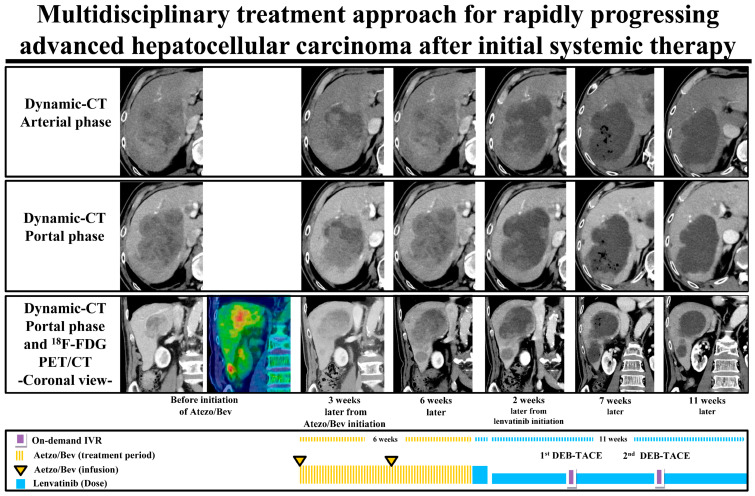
The flow of a multidisciplinary treatment approach for a patient with rapidly progressing advanced HCC after initial systemic therapy. The patient showed rapid progression of HCC after first-line Atezo/Bev therapy and received lenvatinib as second-line treatment. After initiation of lenvatinib, the tumor showed extensive necrosis and good tumor control in combination with subsequent DEB-TACE. Atezo/Bev—atezolizumab plus bevacizumab; DEB—drug-eluting beads; ^18^F-FDG-PET/CT—^18^F-fluorodeoxyglucose positron emission tomography/computed tomography; TACE—transarterial chemoembolization.
